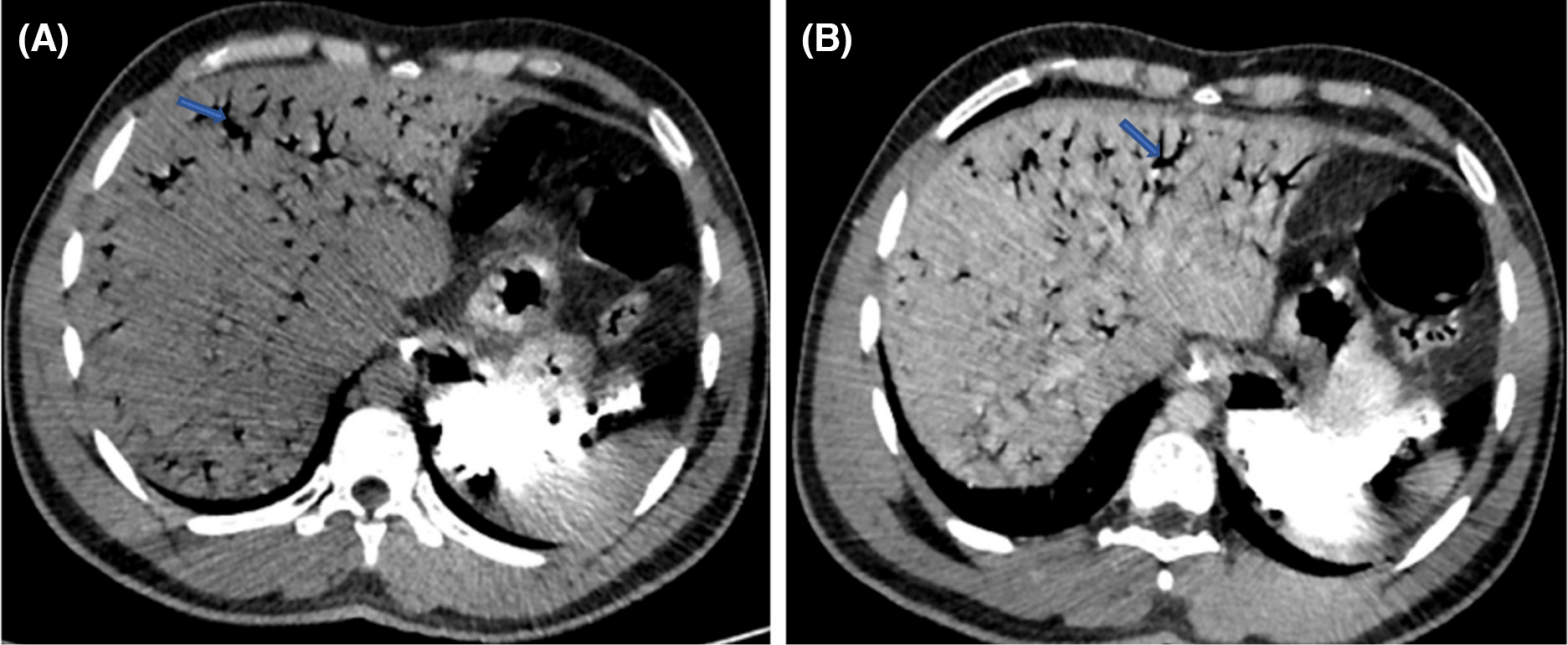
(A): axial noncontrast; (B): axial postcontrast CT images demonstrating linear branching hypoattenuating (HU‐998) areas (arrow) in both lobes of liver extending up to the periphery and in continuation with portal venous branches.
